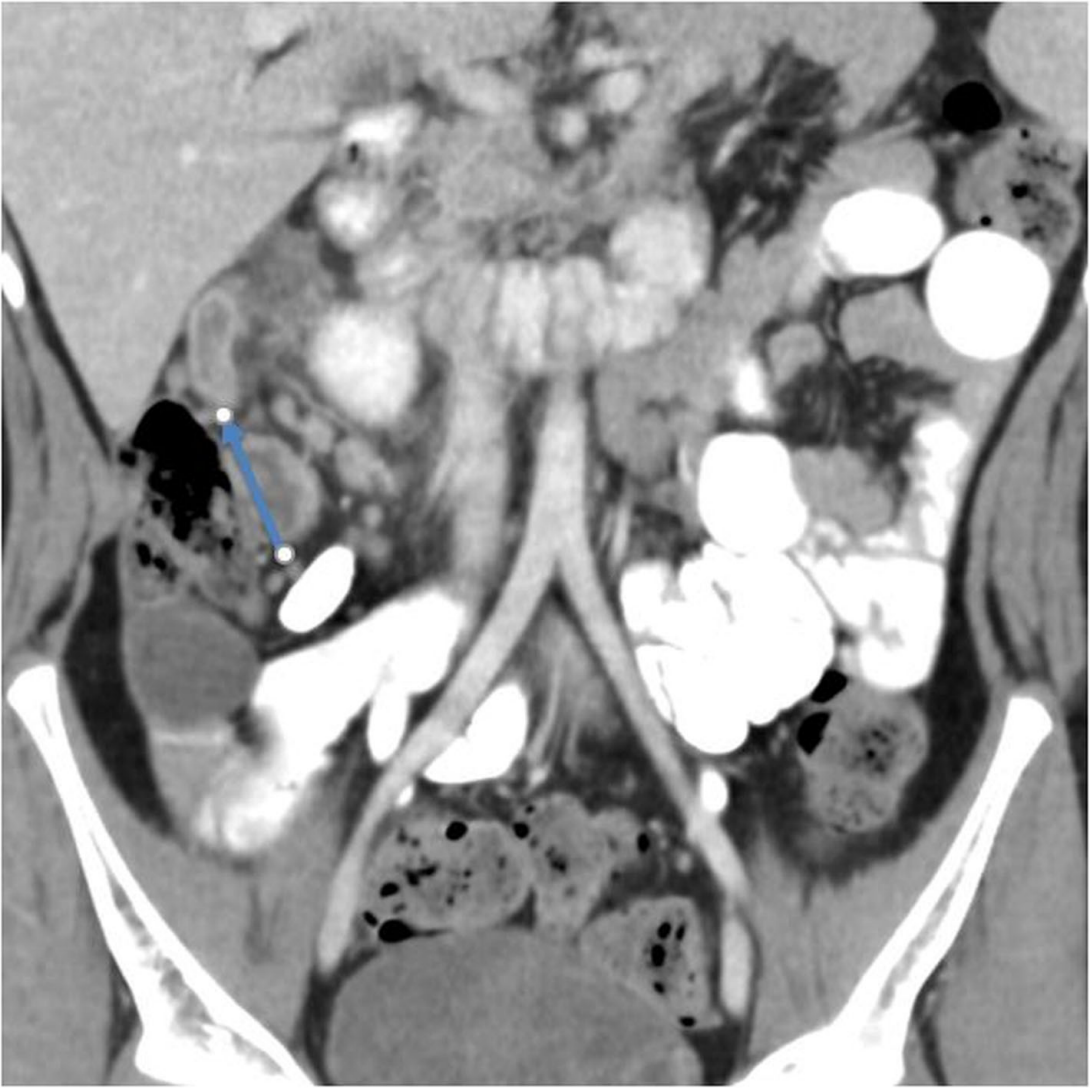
Coronal abdomen CT image (appendicitis protocol) of an 11-year-old male (42 kg) acquired at 6 mGy. Acute appendicitis (arrow) and overall image quality scored optimal (score 3 or 4) by all readers
